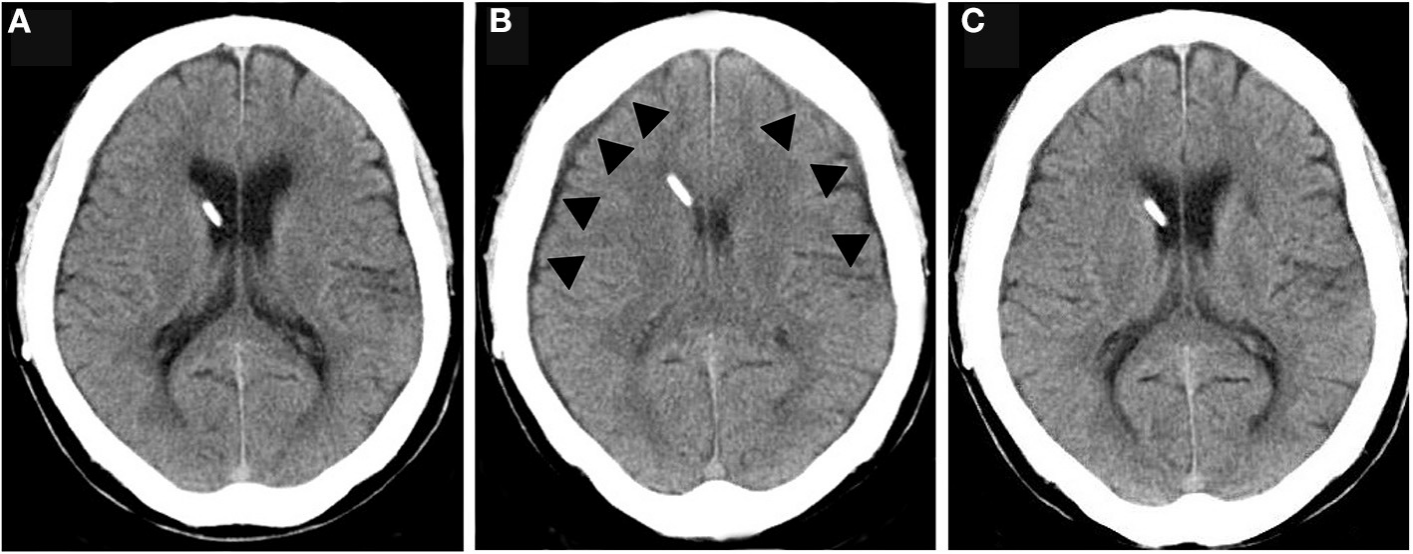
The CSDH caused by overdrainage and disappearance after valve readjustment. (A) CT of normal drainage state after shunting; (B) CT during overdrainage reveal slit ventricle and thin CSDH (arrowhead); (C) CT At 1 month after the valve readjustment shows disappearance of CSDH and slit ventricle.
